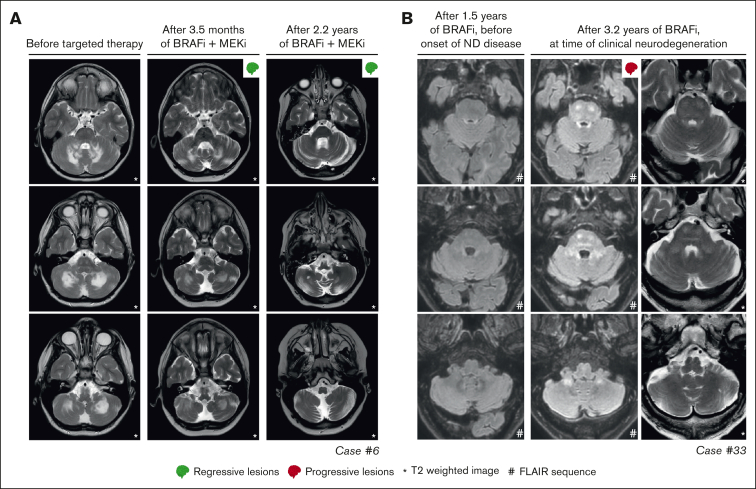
Response and progression of ND lesions. (A) Axial MRI images of case 6, with isolated ND-LCH showing significant reduction in T2-hyperintense lesions after treatment with dabrafenib and trametinib. Note that the remaining hyperintense lesion in the right cerebellum at last follow-up (right column; middle image) is related to the biopsy that was taken from this anatomical site. (B) Axial MRI images of case 33 showing the development of T2- and FLAIR-hyperintense lesions in the pons and cerebellum while receiving treatment with vemurafenib. The patient also developed clinical symptoms of neurodegeneration and died 7.5 months after the MRI scan depicting ND lesions was made. ND, neurodegenerative; FLAIR, fluid-attenuated inversion recovery.
